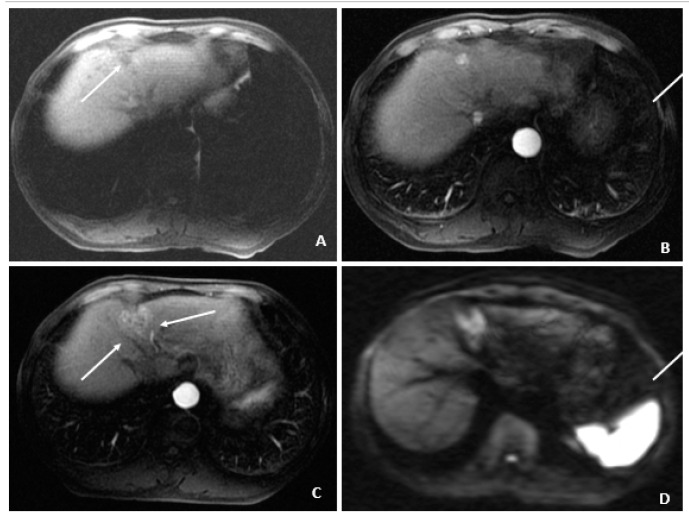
Recurrent HCC after cryoablation (August 2009 and July 2010). (A) At 3 months following cryoablation, the axial T1-weighted fat-suppressed precontrast image shows a small hypointense lesion (arrow) above and in proximity to the recently ablated HCC. (B) The corresponding arterial-phase postcontrast image shows hyperenhancement (arrow), but additional features (i.e., washout, capsule appearance, etc.) were not observed. (C) Months later, a follow-up MRI showed threshold growth of the hyperenhancing lesion (arrows). (D) The corresponding diffusion-weighted image shows diffusion restriction (arrows) and helps to confirm the diagnosis of HCC (as an ancillary feature); washout was also evident (not shown), confirming the diagnosis of HCC.
